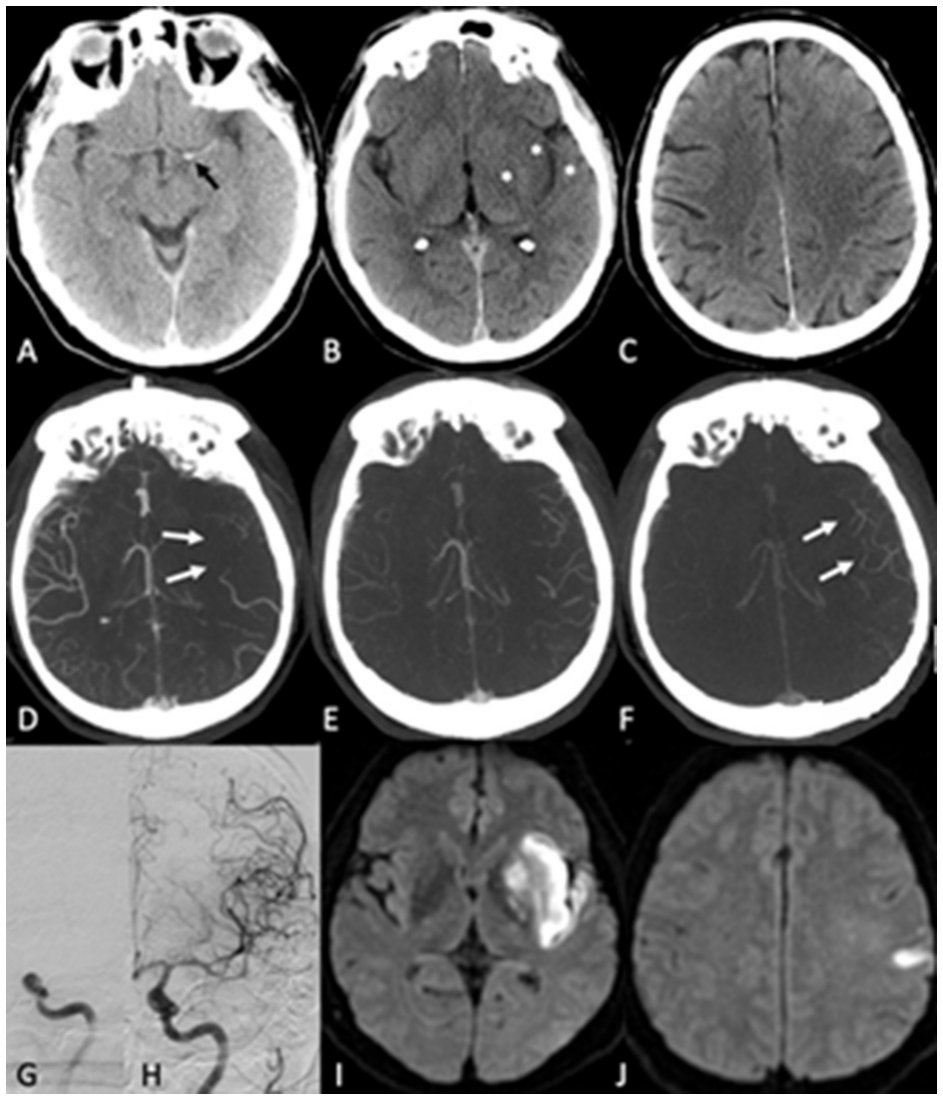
Acute ischemic stroke due to a terminal internal carotid artery occlusion. (A,B) show the initial Plain CT. A hyperdense vessel sign [black arrow in (A)] is seen, and there is loss of gray-white matter differentiation in the left insula, lentiform nucleus and M2 region [asterisks in (B)], corresponding to an ASPECTS score of 7. No ischemic changes are seen at the supraganglionic level (C). Multiphase CTA shows lack of collateral filling in the first (arterial) phase [white arrows in (D)]. However, the pial arterial collaterals fill eventually in the second (peak-venous) phase (E). There is a slight delay in washout in the third phase (F). Intravenous thrombolysis was administered between Plain CT and mCTA, and the patient was treated with EVT after mCTA has been completed. (G) shows the initial digital subtraction angiography run with the occlusion. (H) shows the last intracranial angiography run with complete recanalization [modified thrombolysis in cerebral infarction (mTICI 3)]. On follow-up diffusion-weighted MRI at 24 h, an infarct of moderate size in the left insula, lentiform nucleus, and M2 region was seen (I), corresponding to the areas with early ischemic changes in the initial plain CT. There was also a small infarct in the left M5 region (J) that was not noted on the baseline plain CT.
